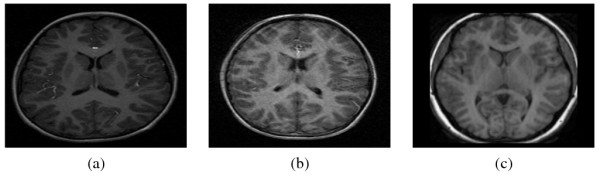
Sample control and ADHD MRIs from URNC and IBSR databases. Control (a) and ADHD (b) example MRI slices from the URNC database and an example MRI slice from the IBSR database (c).
